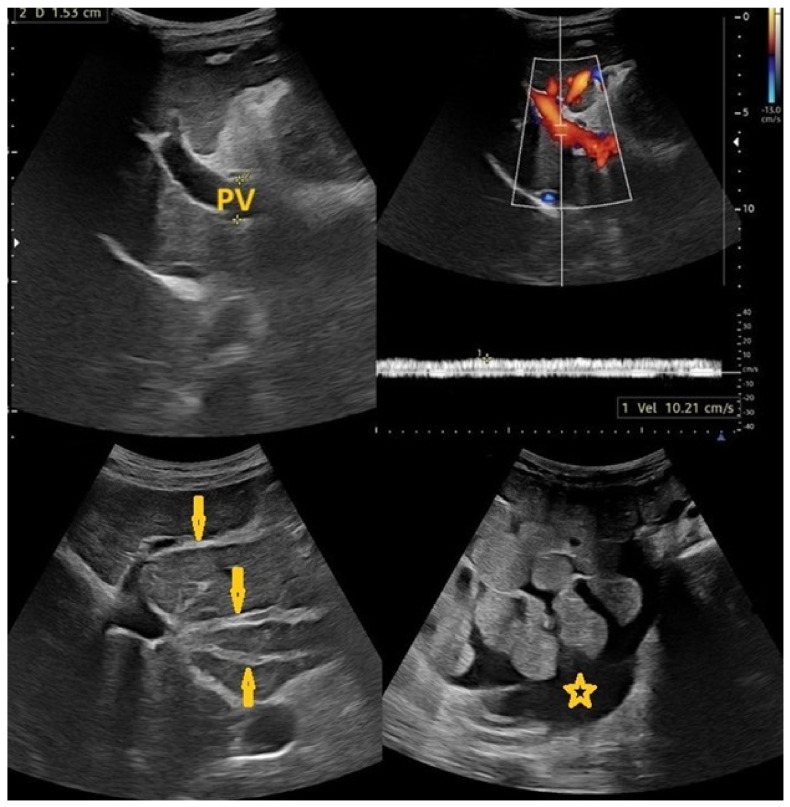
Selected sections of ultrasonography of a 32-year-old woman’s abdomen show an average-size liver with irregular borders, coarse texture, periportal fibrosis (arrows), and signs of portal hypertension, including the following: ascites (star) and splenomegaly (up to 15 cm in craniocaudal diameter, portal vein diameter of 14 mm, hepatofugal flow with reduced velocity in PV to 12 cm/s, with portosystemic collateral veins in the epigastric and splenic hila). Picture of liver cirrhosis with portal hypertension and complicated chronic Schistosomiasis.
